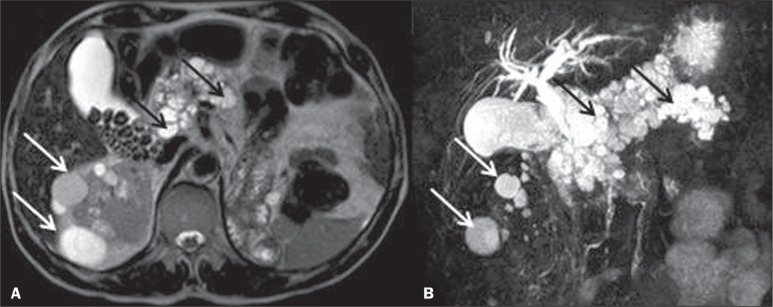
MRI. Axial T2-weighted sequence (A) and three-dimensional cholangiography
